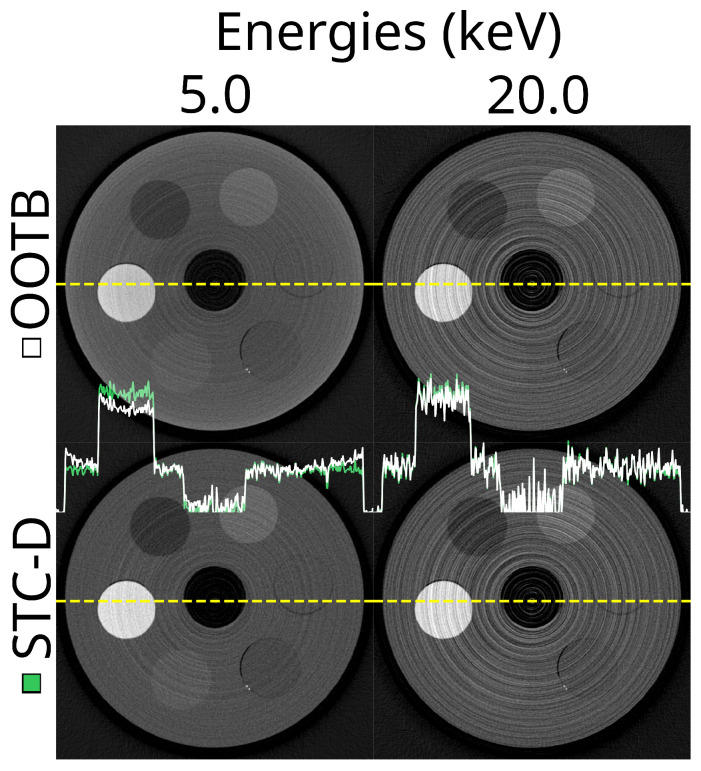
These are the results of applying a beam hardening correction method (STC-D) to CT reconstructions. The top row shows reconstructed slices at 5.0 keV and 20.0 keV without any beam hardening correction (out-of-the-box, OOTB). The bottom row shows the same reconstructions after applying the STC-D beam hardening correction. In the centre of the image are profile plots. These are the profiles of the uncorrected (white, OOTB) and corrected (green, STC-D) reconstructions along the yellow dotted lines. The detector was not moved; no DAC-shifting or any form of STC was used, while FDK was used for reconstruction. This uses the ASI detector (‘ASI-1’ data). The beam hardening correction (STC-D) visibly reduces artefacts in the reconstructed images compared to the out-of-the-box (OOTB) results.
